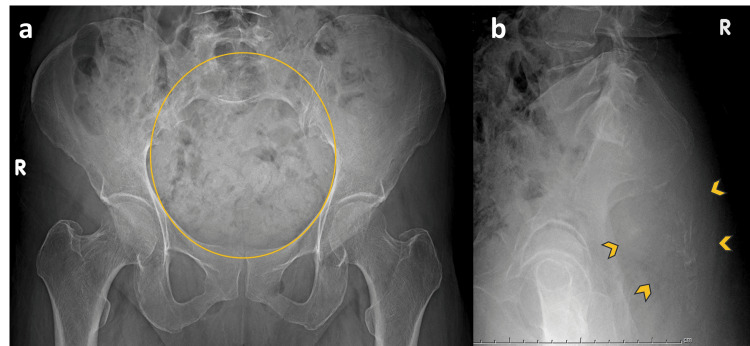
Subtle radiographic findings of sacral chordoma Anteroposterior (a) and lateral (b) pelvic radiographs demonstrate non-visualization of the distal sacral segments (circled area) with subtle loss of normal sacral contour. Ill-defined soft tissue fullness is seen in the sacral and presacral region (arrowheads). Findings are inconspicuous, highlighting the limited sensitivity of plain radiography.
